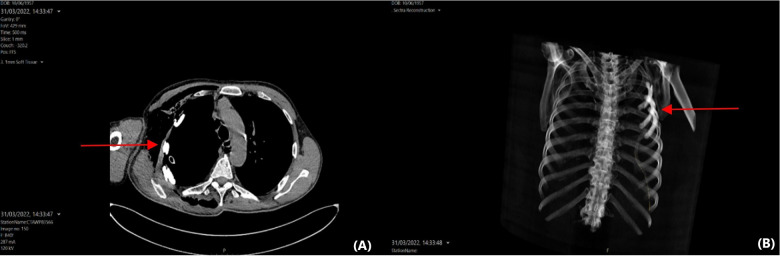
(A) Axial computed tomography of the thorax showing a personalised prosthesis contoured to the concavity of the chest wall and residing anatomically. (B) 3D reconstruction of the same computed tomography scan.
